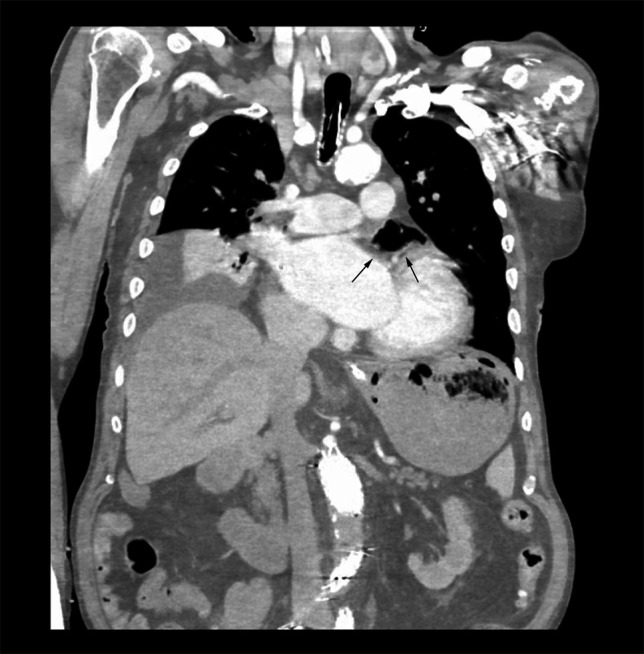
Computed tomography angiogram of the chest and abdomen demonstrated large amounts of air in the left atrium and small amounts in the left ventricle and right atrium.
